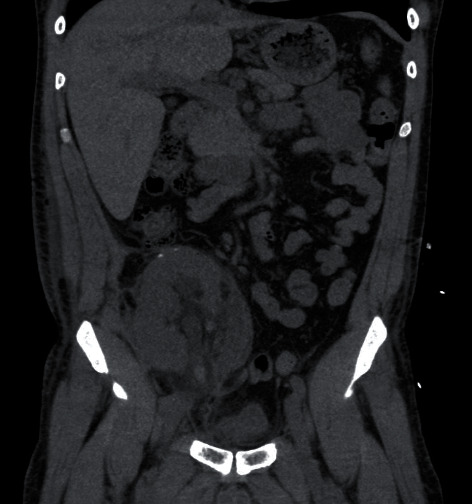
Coronal view of the CT scan showing hydronephrosis and clot in the transplanted ureter.
